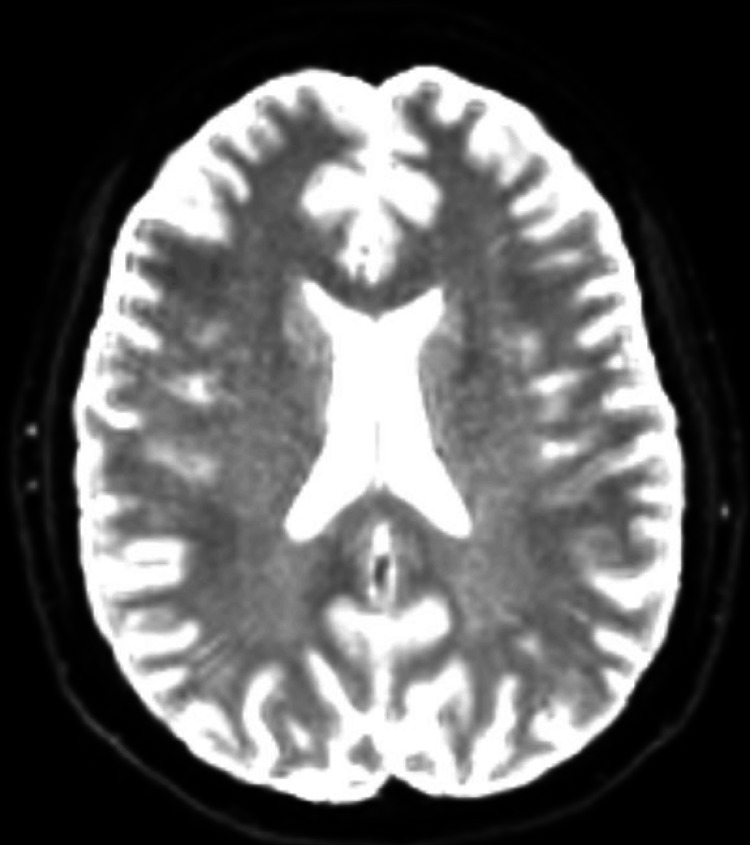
Diffusion-Weighted Imaging (DWI) Sequence MRI of the Brain Diffusion-weighted imaging MRI of the brain showing no evidence of acute pathology, including no restricted diffusion to suggest acute infarction.
